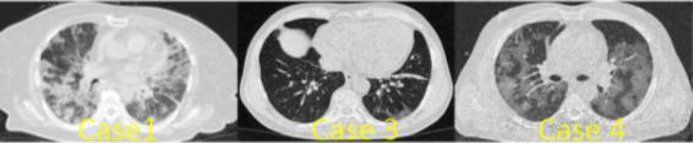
Bilateral ground-glass opacification in lung CT in cases 1, 3, and 4
